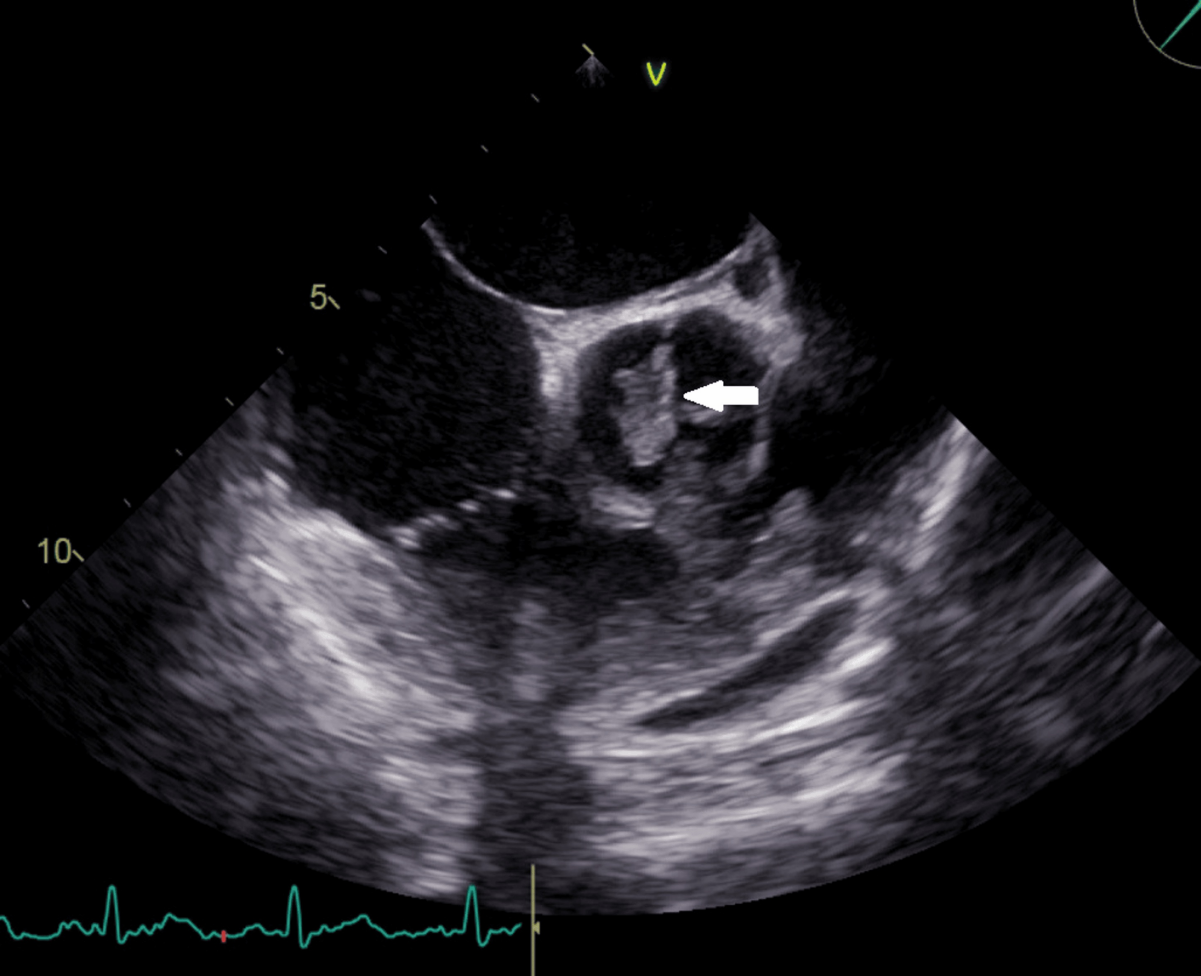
Transesophageal echocardiogram showing a large vegetation (white arrow) over the aortic valve
